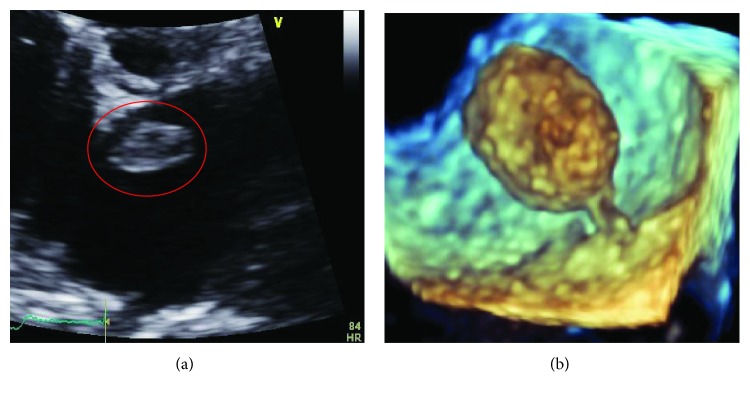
Transthoracic parasternal short axis (a) and transesophageal 3-D (b) images of pedunculated left atrial mass.
